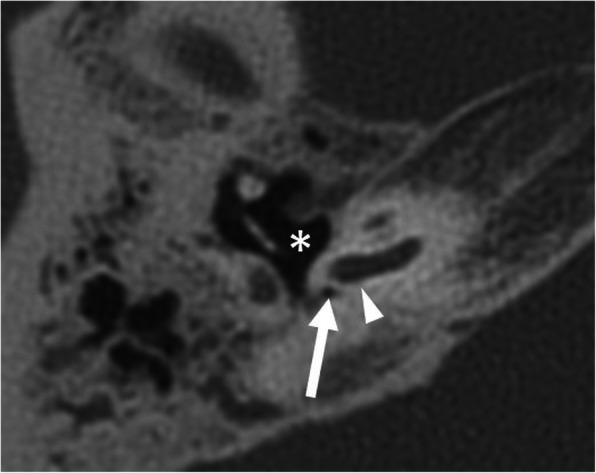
A 65-year-old male patient, with external auditory canal hypoplasia and bilateral SNLH from birth. HRCT axial image shows a hypoplastic round window (arrow) and a small tympanic cavity (asterisk). This condition complicates anatomical orientation and surgical access to the basal turn (arrowhead)
